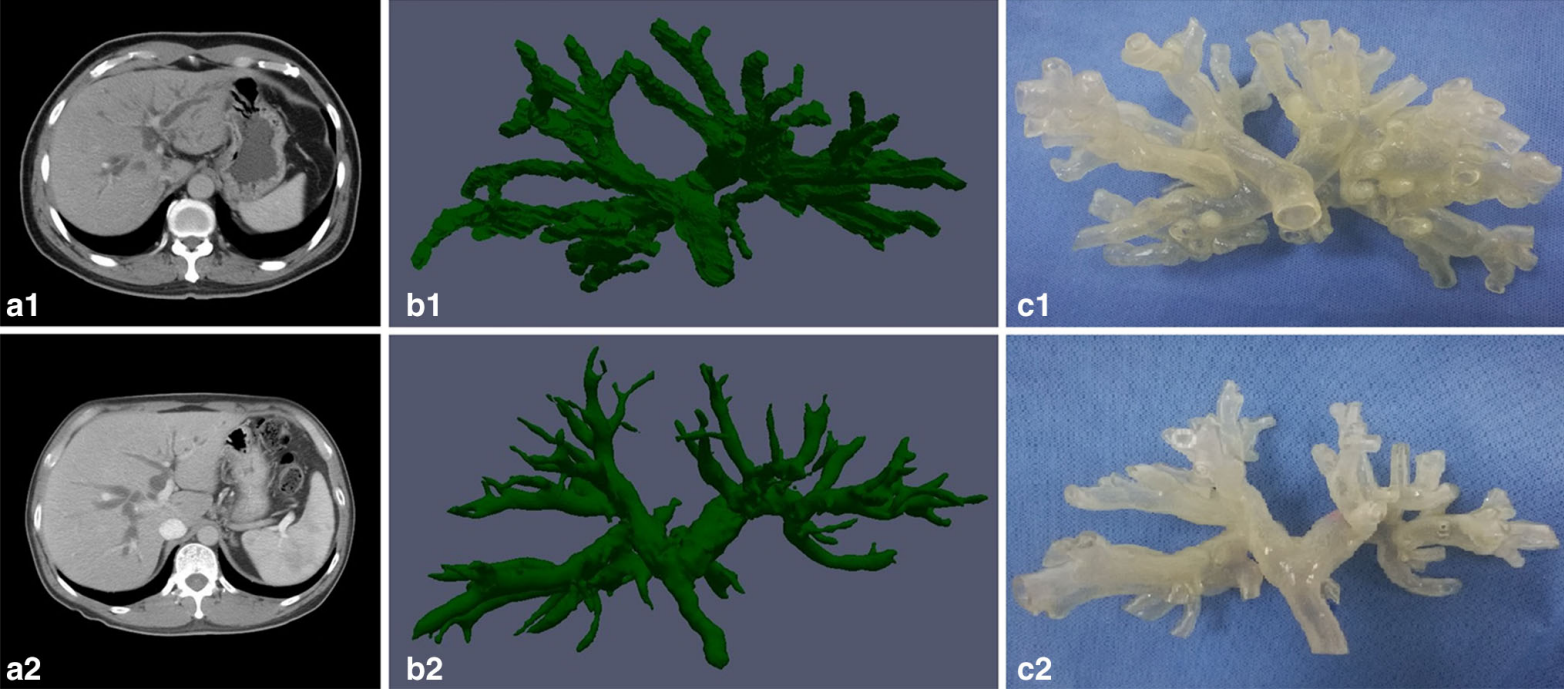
Computed tomography (CT) images (a1, a2), three-dimensional (3D) reconstruction (b1, b2) and three-dimensional printing (3DP) (c1 Model No. 1, c2 Model No. 2) of two patients with biliary tract dilatation
